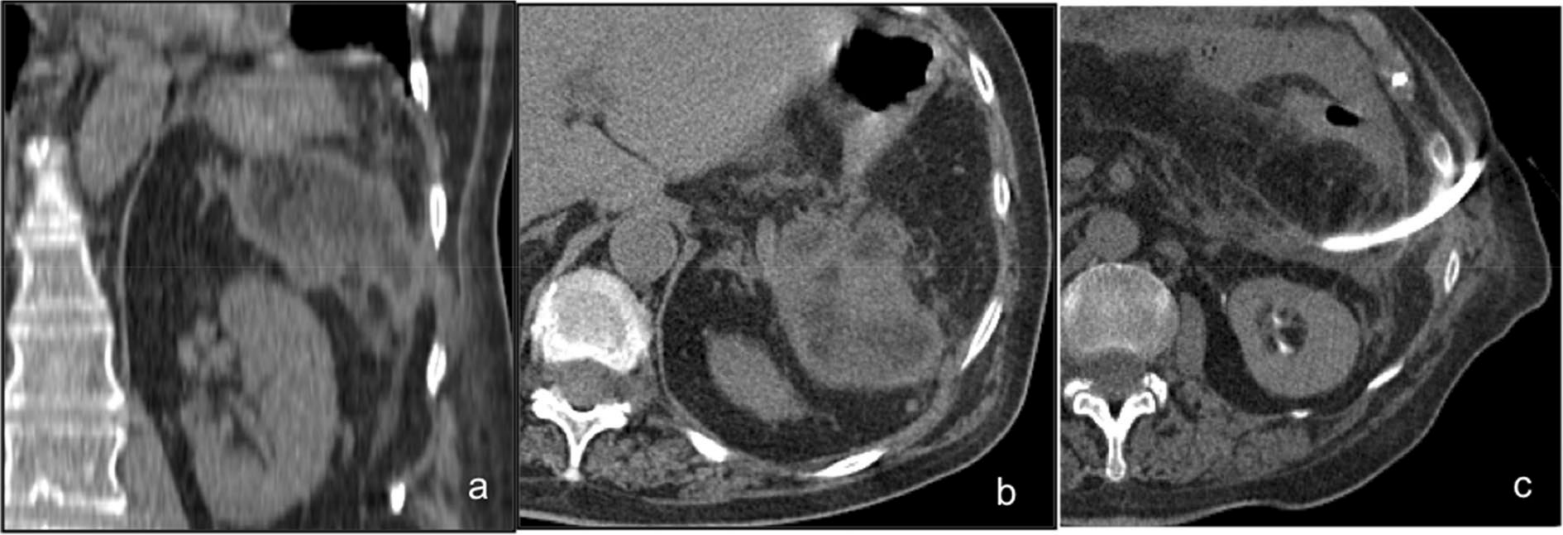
Coronal (a) and axial (b) CT scans of a 72-year-old patient with left subphrenic abscess after spleno-pancreatico-duodenectomy, drained with a 12F PIGTAIL catheter (c)
